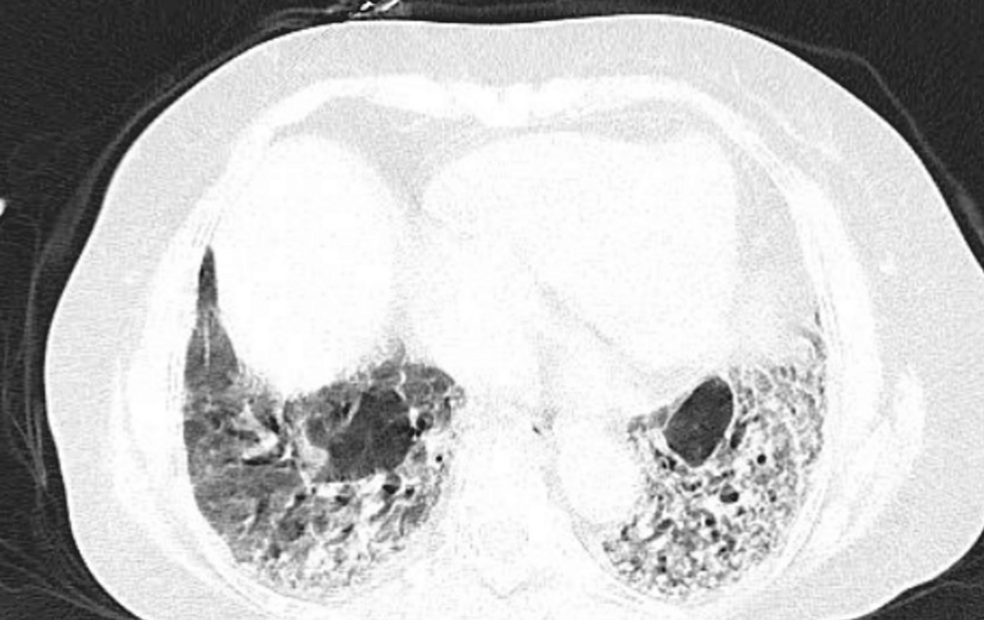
This is a thoracic HRCT that showed several “honeycomb” lesions in both lower lobes. Additionally, there were two characteristics that are indicative of subsequent ILD: intralobular septal thickness and glasses haziness. HRCT, high-resolution computed tomography; ILD, interstitial lung disease
